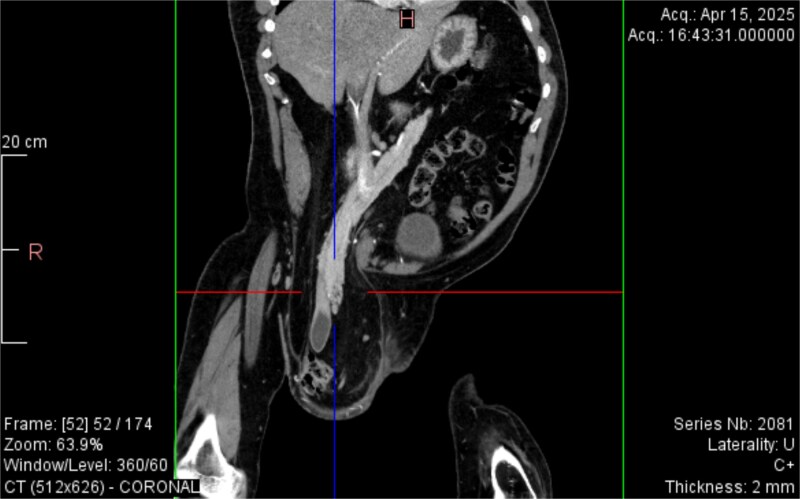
Coronal CT view inguinal hernial sac with pancreatic head and D2 and D3 segment of duodenum.
